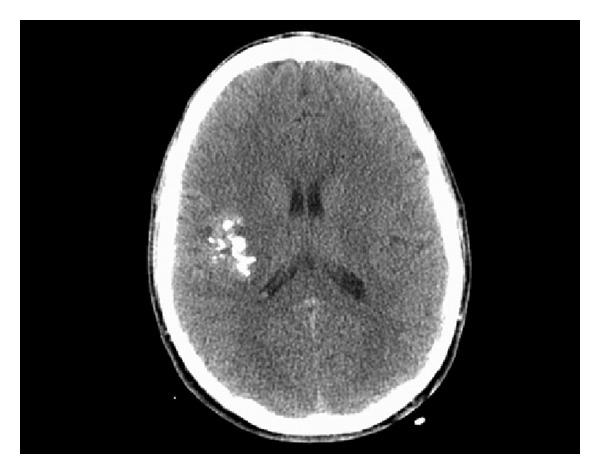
Axial CT without contrast demonstrates right posterosuperior temporal lobe ill-defined clumped calcification.
